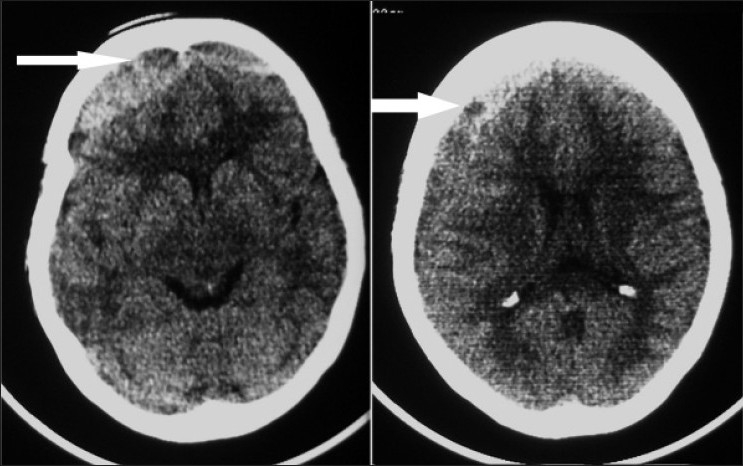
Computed tomogram without contrast, showing large bifrontal hyperdense convex-shaped collection (left), also associated crescent-shaped collection over right frontoparietal region (right) (note - both the frontal horns of lateral ventricles are widely displaced laterally and pushed posteriorly), also note inhomogeneous nature of the hematoma (a tendency of liquefaction of the blood on the right high parietal region-arrows)
